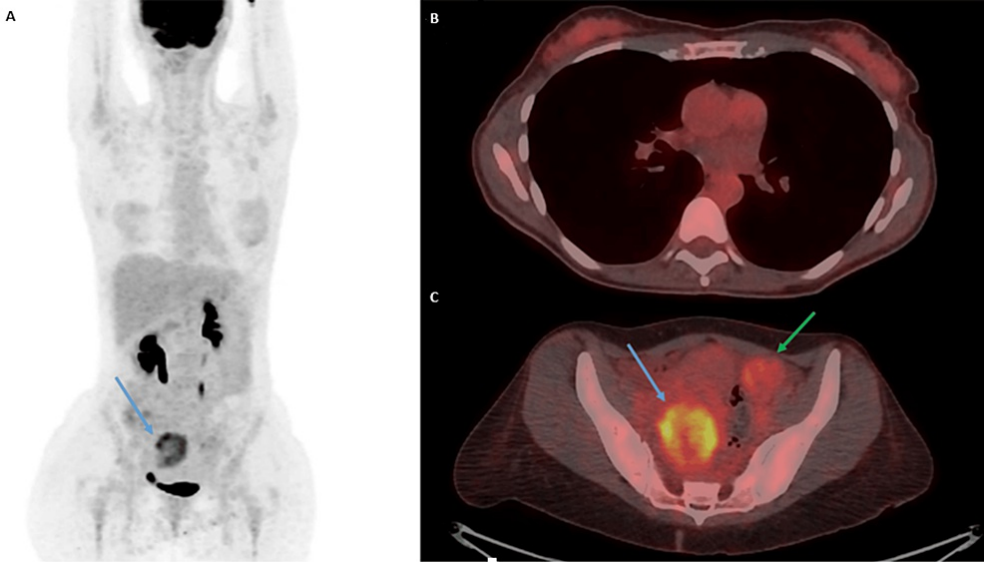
Positron emission tomography/computed tomography The maximum intensity projection image shows only a significant uptake in the right pelvic region (panel A, blue arrow). Axial positron emission tomography/computed tomography images show a mild diffuse uptake in the bilateral mammary gland without observing clear pathological foci, probably physiological (panel B). Axial positron emission tomography/computed tomography image in which a moderated uptake is identified in the right ovarian lesion (blue arrow), observing a lesser uptake in the left ovarian lesion (green arrow), similar to the surrounding background activity (panel C).
